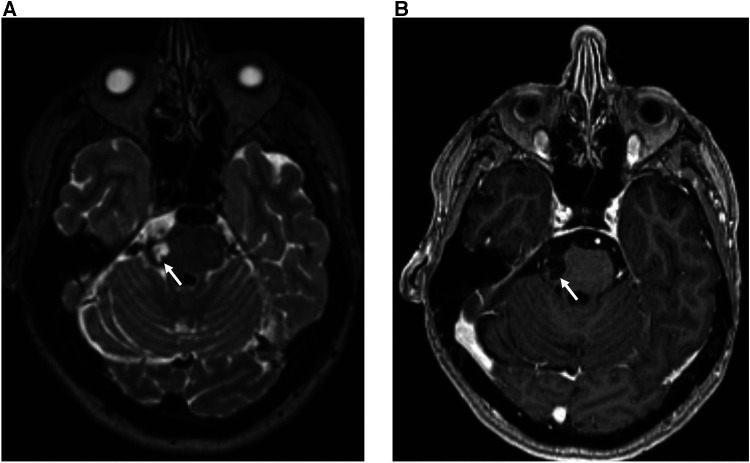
30 year-old female with sudden onset headache and right-sided facial sensory disturbance. MRI head study performed several days after initial symptom onset demonstrates a subacute haematoma on the lateral aspect of the right ventral pons at the site of entry of the right trigeminal nerve characterized by: (A) high T2 signal relative to grey matter on the axial T2-weighted sequence (white arrow) (B) low T1 signal relative to grey matter and absence of contrast enhancement on the gadolinium-enhanced axial T1-weighted sequence (blue arrow). The patient was subsequently found to have a small arteriovenous malformation on digitally-subtracted cerebral angiography (not shown).
